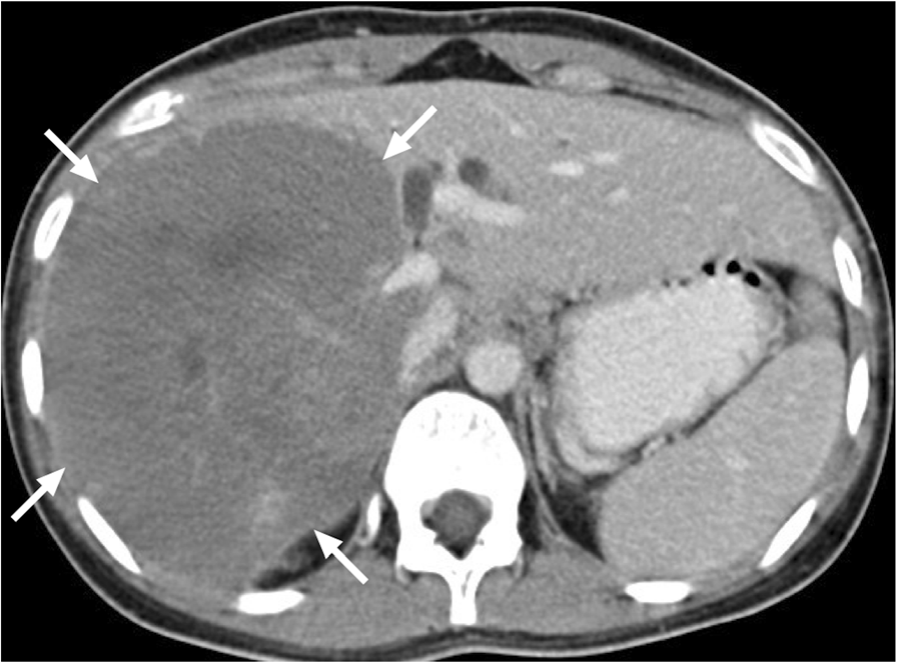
A 33-year-old female with no known past medical history presented with gradually increasing right upper quadrant pain. Abdominal postcontrast CT demonstrates a hypoattenuating centrally necrotic massive mass lesion (arrows). There was no evidence of obvious enhancement after contrast injection. Percutaneous biopsy revealed alveolar hydatid cyst
